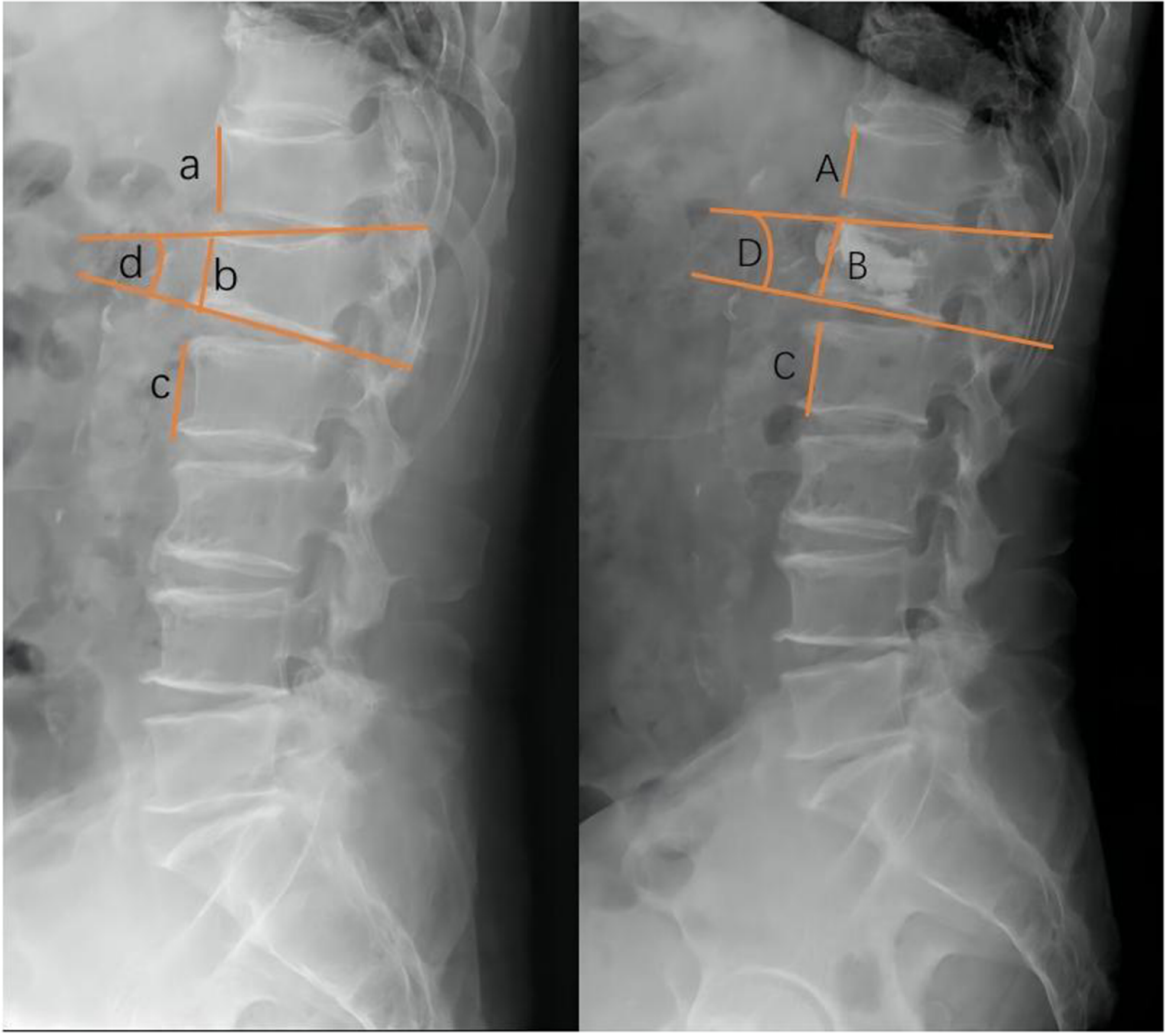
Radiographic evaluation of compressed vertebrae. Preoperative VHR (left,%):2b/a + c, VKA (left,°):∠d; Postoperative VHR (right,%):2B/A + C, VKA (right,°):∠D.
